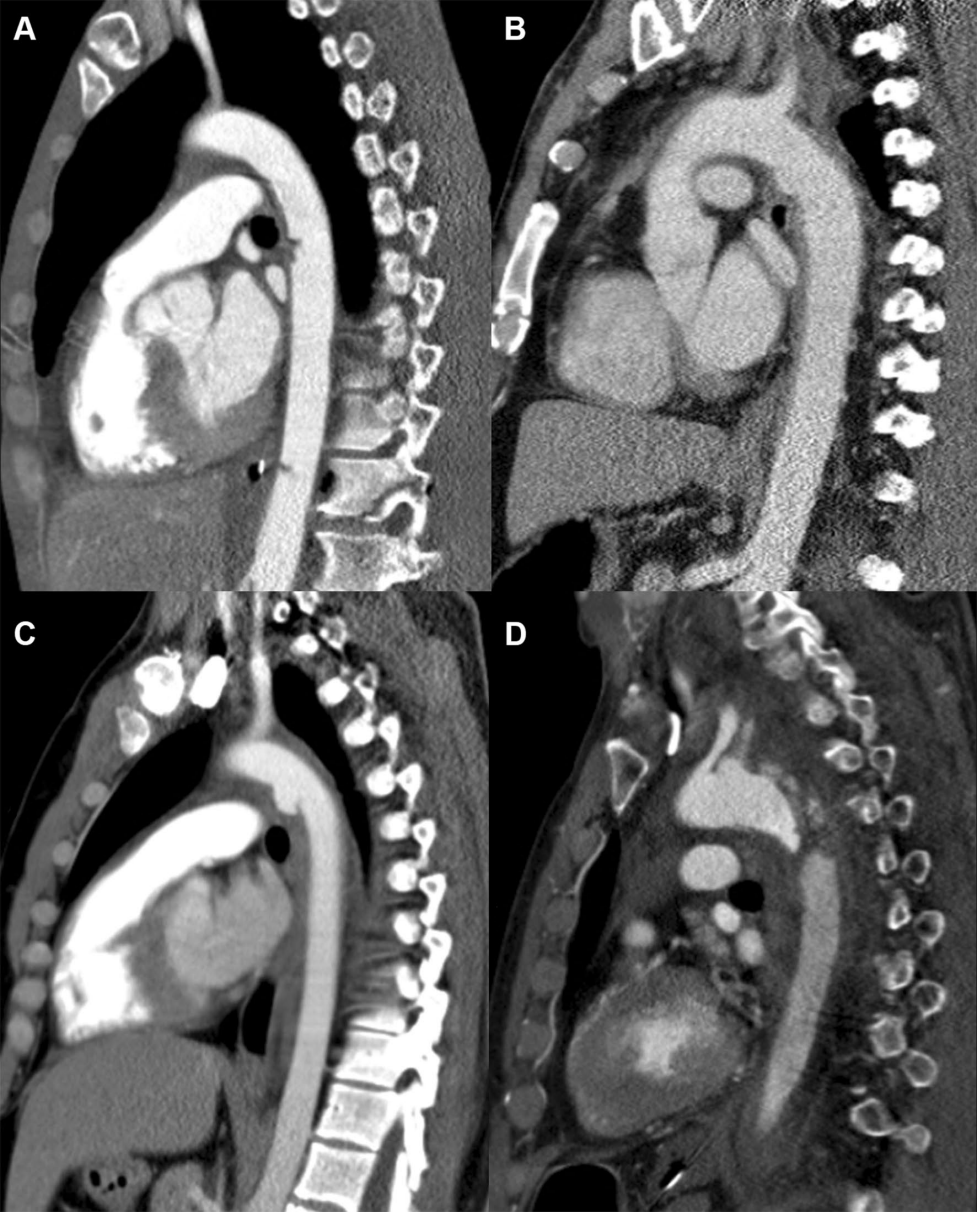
Computed tomography angiography images of the four Azizzadeh et al. blunt thoracic aortic injury (BTAI) grades. a Grade I, intimal tear: two intimal tears of the descending thoracic aorta are visible. b Grade II, intramural hematoma: distal side of the aortic arch. c Grade III, pseudoaneurysm: distal to the left subclavian artery. d Grade IV, rupture: aortic arch disruption causing massive hemothorax resulting in decreased contrast enhancement of the descending aorta
